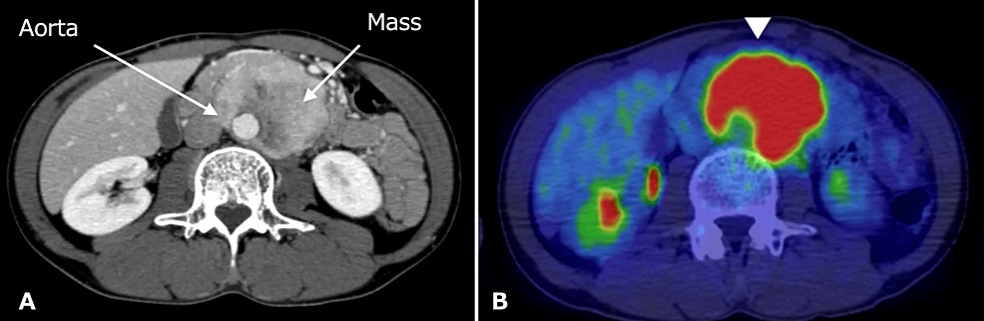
Preoperative CT findings Contrast-enhanced CT revealed a retroperitoneal mass of about 70 millimeters surrounding the abdominal aorta (A). PET scan showed a significant accumulation of the abdominal mass (arrowhead) (B).
